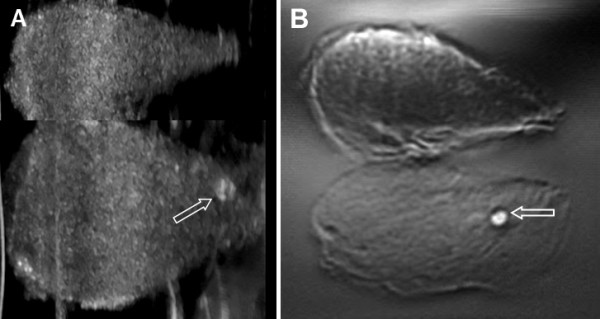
Excised Human Thyroid. A) C-mode ultrasound of an excised human thyroid from a cadaver, B) VA image of this tissue in 60 × 60 mm scan dimension. The arrow shows a 2.5 x 2.5 mm calcification in VA image with more contrast than shown in the US image.
